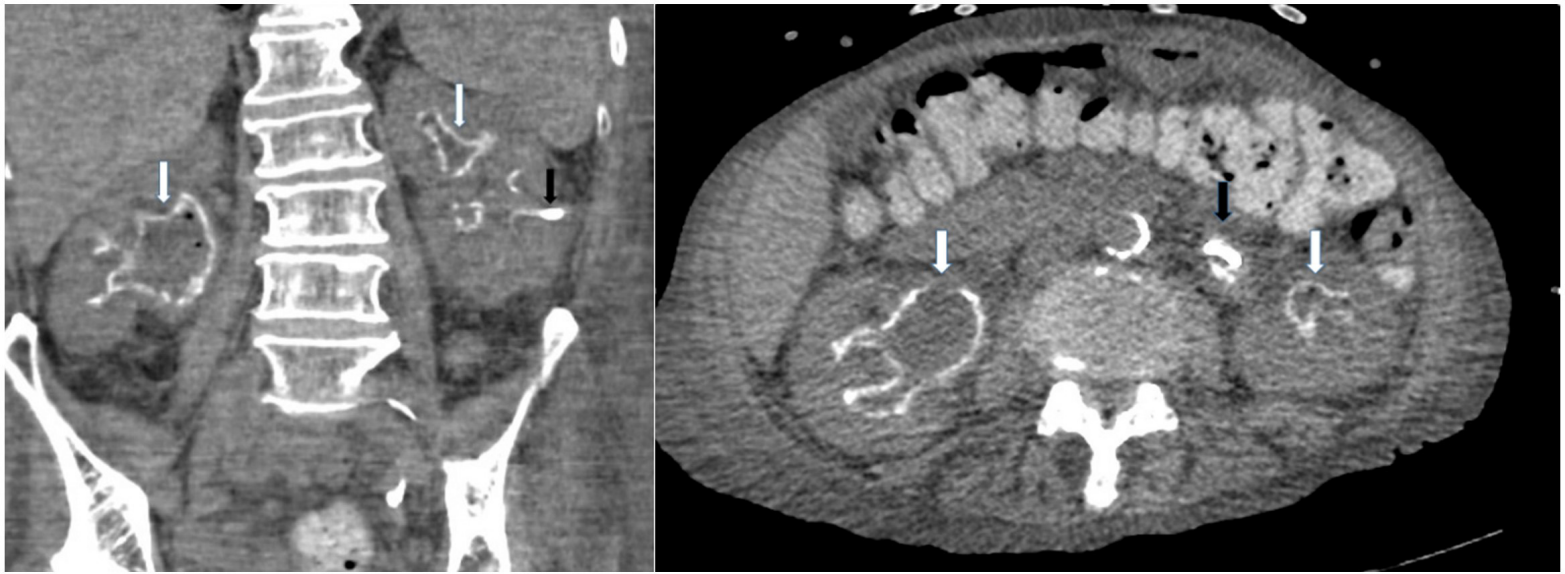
Non-enhanced CT-scan showing encrusted pyelitis. White arrow: linear calcifications of the renal collecting system with bilateral ureterohydronephrosis, black arrow: nephrostomy catheter.
